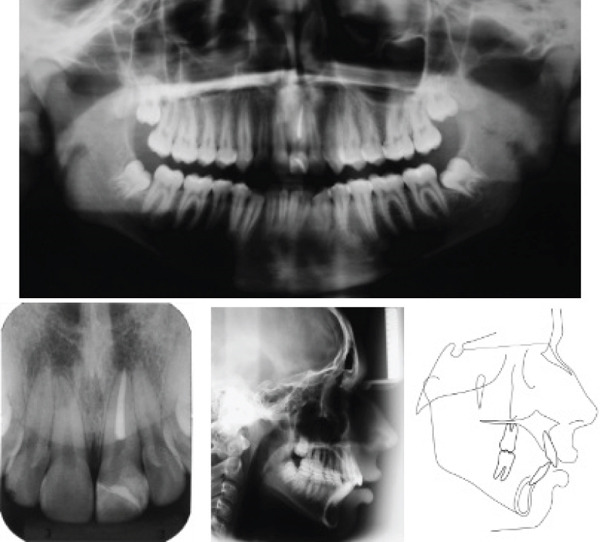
Pretreatment panoramic, periapical, and lateral cephalometric radiographs and tracing.
